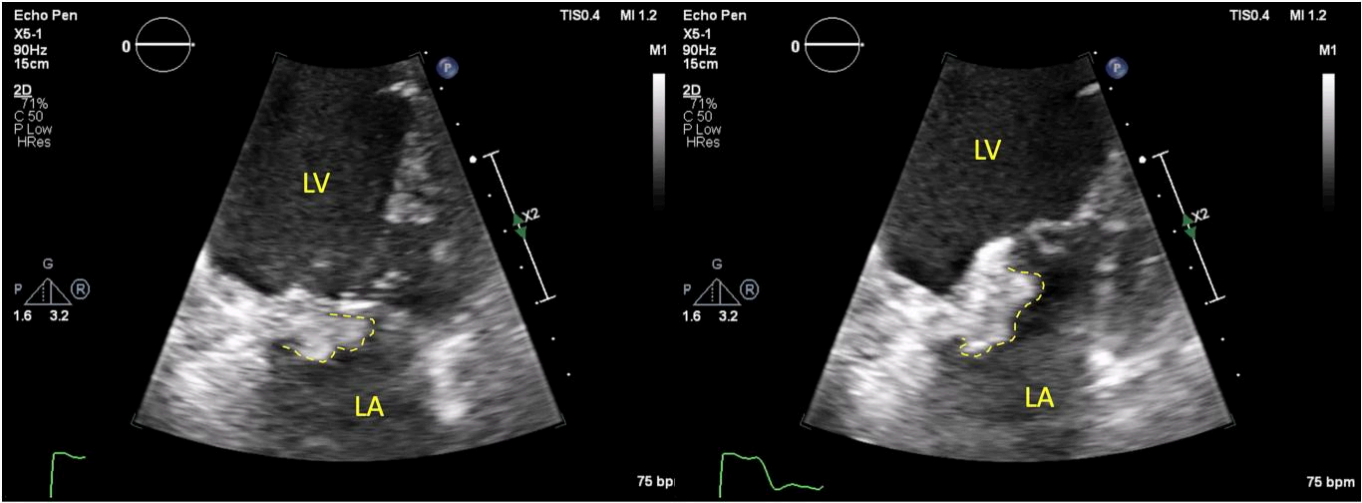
Transthoracic echocardiography, two-chamber view, zoom. On admission during the second hospitalization in 2021. Yellow line shows vegetation on leaflets of the mitral valve. LA, left atrium; LV, left ventricle.
